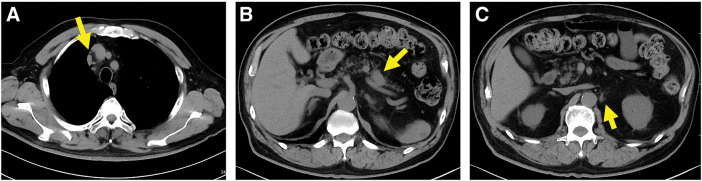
Axial view on computed tomography after 6 courses of pembrolizumab therapy. (A) The anterior mediastinal lymph node shrank (3.0 mm) (arrow). (B) The primary tumor shrank (26.3 mm) (arrow). (C) The para-aortic lymph node shrank (3.0 mm) (arrow).
